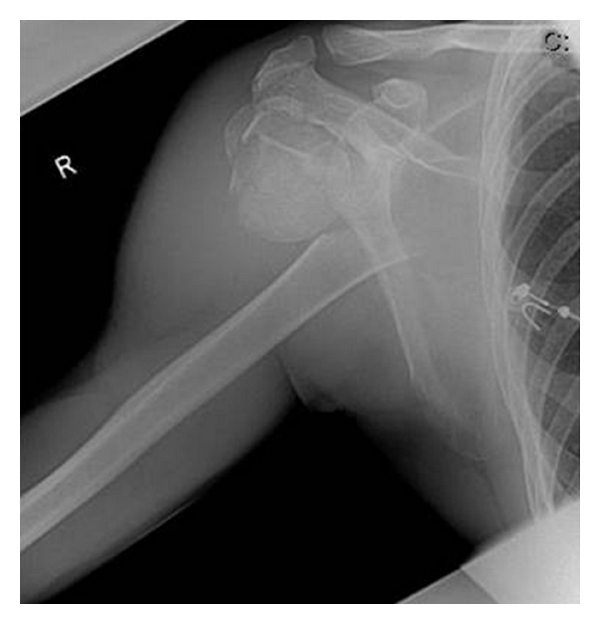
Initial X-ray of the dislocated proximal humeral fracture.
